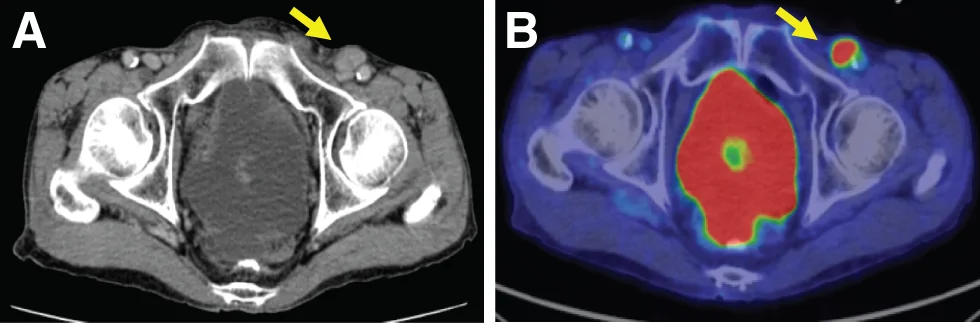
Findings of contrast-enhanced CT and PET/CT. (A) Contrast-enhanced CT revealed an enlarged lymph node in the left inguinal region (yellow arrow). (B) PET/CT scan revealing fluorodeoxyglucose uptake in the left inguinal lymph node only (yellow arrow).
